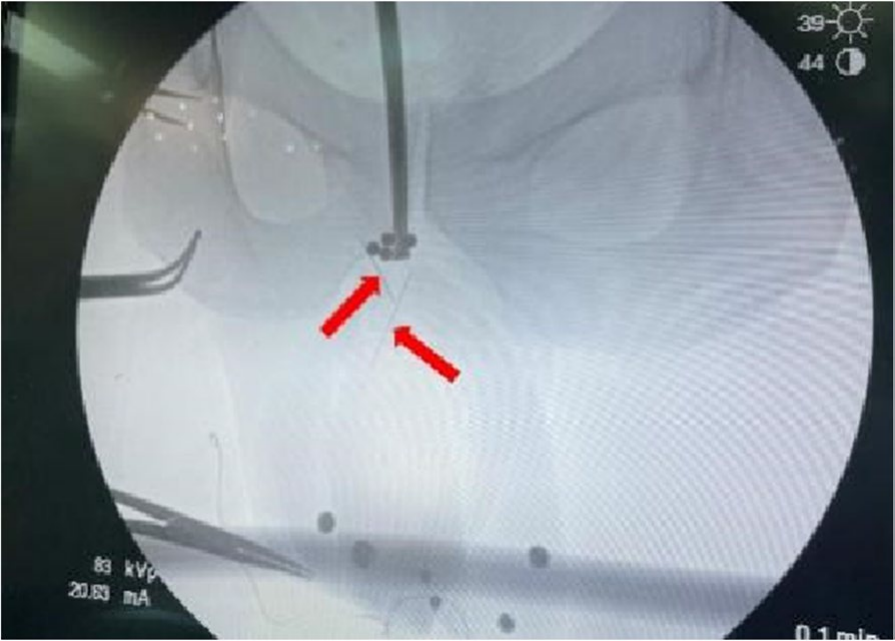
Intraoperative X-ray of the patient’s first surgery on July 26, 2023. The arrows show the needles
